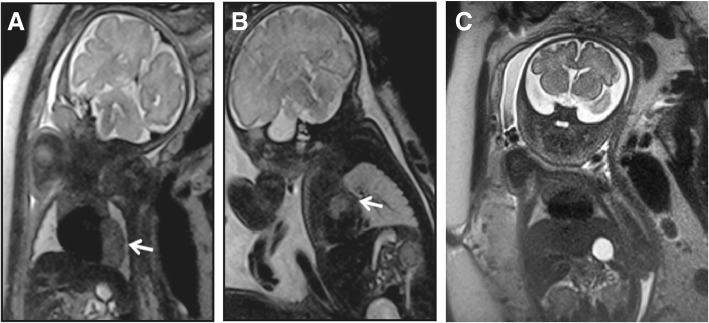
Use of MRI for the diagnosis of suspected fetal congenital heart disease in pregnant women. Fetal MRI revealed (a) T2-hypointense lesions on the left ventricular free wall and (b) lesions on the left atrium in a case of suspected fetal congenital heart disease. Lesions were indicated by white arrows. (c) Fetal MRI of a normal fetus as a control. All images were acquired using T2-Haste sequence using the following parameters: TR = 1000 ms, TE = 92 ms, slice thickness = 4 mm
